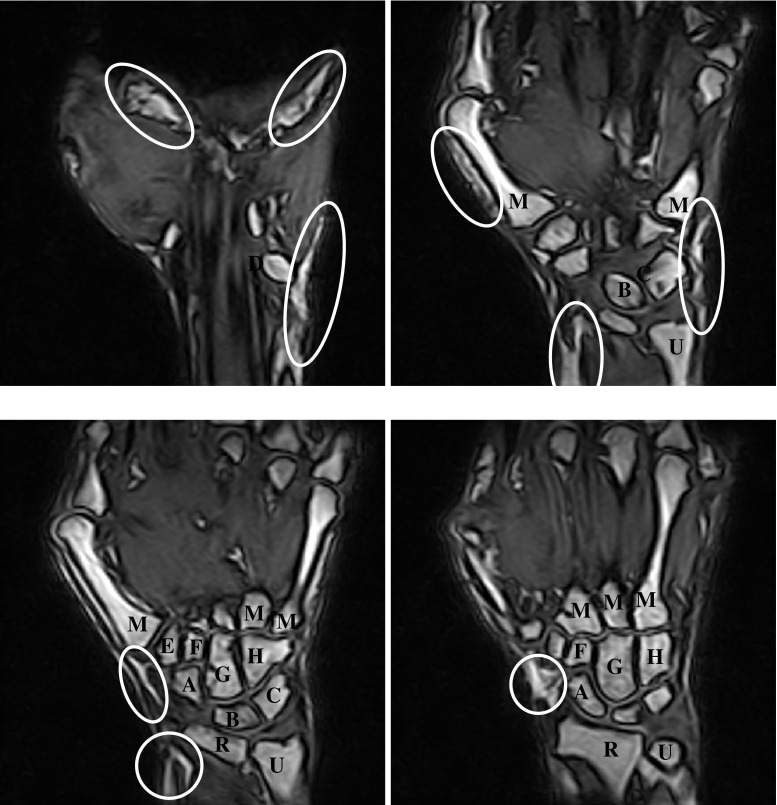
Four coronal slices of MR image of a wrist. *Ellipses* surround soft tissue regions characterized by the same signal intensity range as wrist bone interiors. In the slices, wrist bones are marked: *R* radius, *U* ulna, *M* metacarpals, *A* scaphoid, *B* lunate, *C* triquetrum, *D* pisiform, *E* trapezium, *F* trapezoid, *G* capitate, *H* hamate
